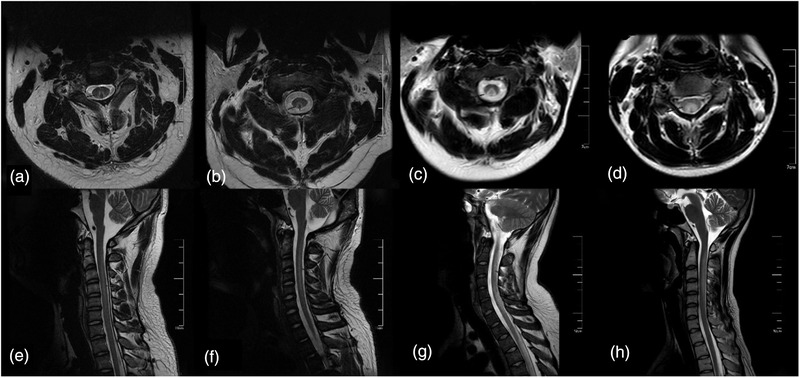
Spinal magnetic resonance imaging. (a–d) Transverse sections of spinal cord showing “V,” inverted “V,” triangle, and elliptical signals. (e–h) Sagittal sections showing high T2 signal lesion involving the dorsal longitudinal segment of the spinal cord
